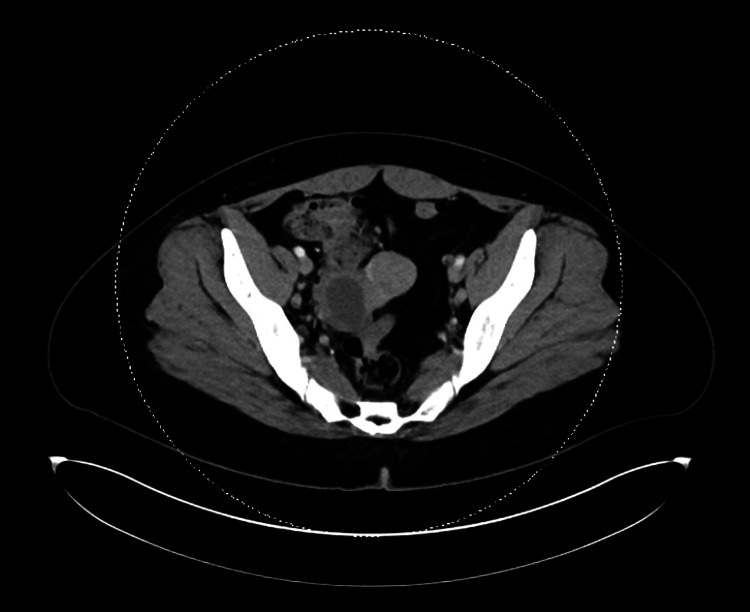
CT CAP with contrast showing right common iliac lymphadenopathy CAP: chest, abdomen, and pelvis; CT: computed tomography
